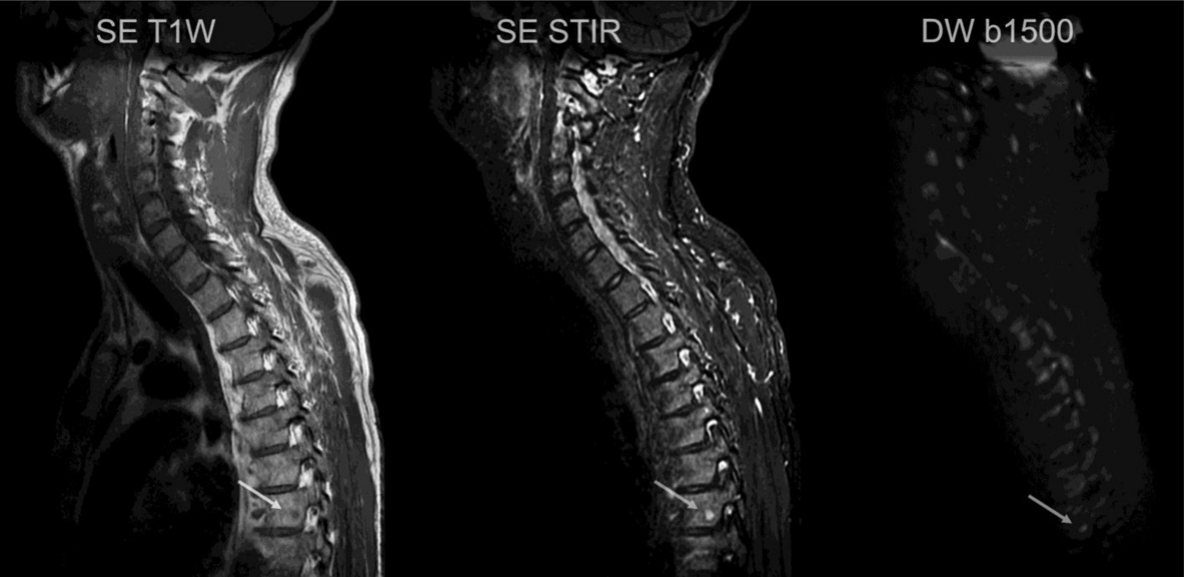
Magnetic resonance imaging visualizing metastatic spine lesion (*arrow*)
